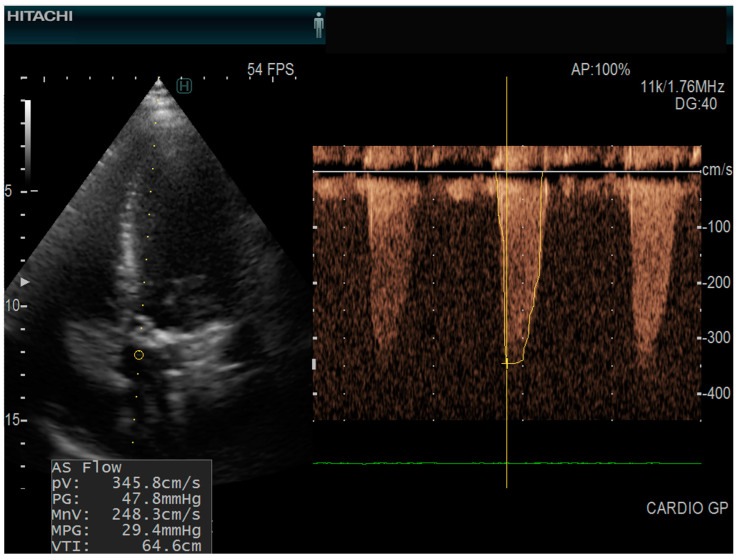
Echocardiography of a patient with aortic stenosis echocardiograph: Hitachi Arietta 65, apical five chamber view, continuous pulse wave doppler AS Flow = aortic stenosis flow; (PV = peak velocity; PG = peak gradient; MnV = mean velocity; MPG = mean pressure gradient; VTI = velocity time integral).
